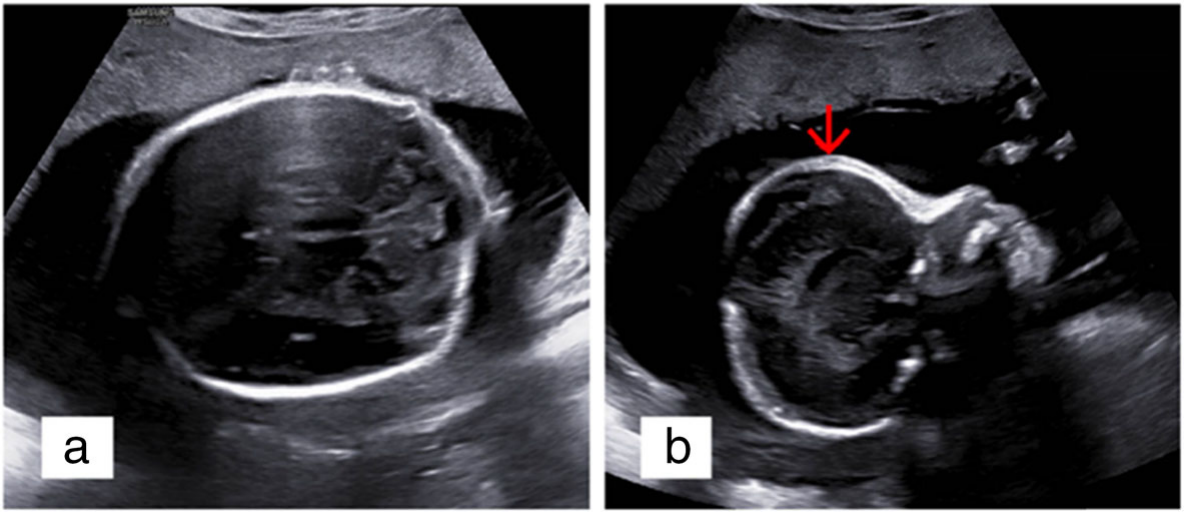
Indirect signs of craniosynostosis showed by 2D prenatal ultrasound scanning. a brachycephaly with an 77% CI, absence of lucency at the coronal suture, and the brain shadowing sign were showed in fetal cross-section. b Turribrachycephaly was showed in sagittal section. Red arrow: frontal bossing
